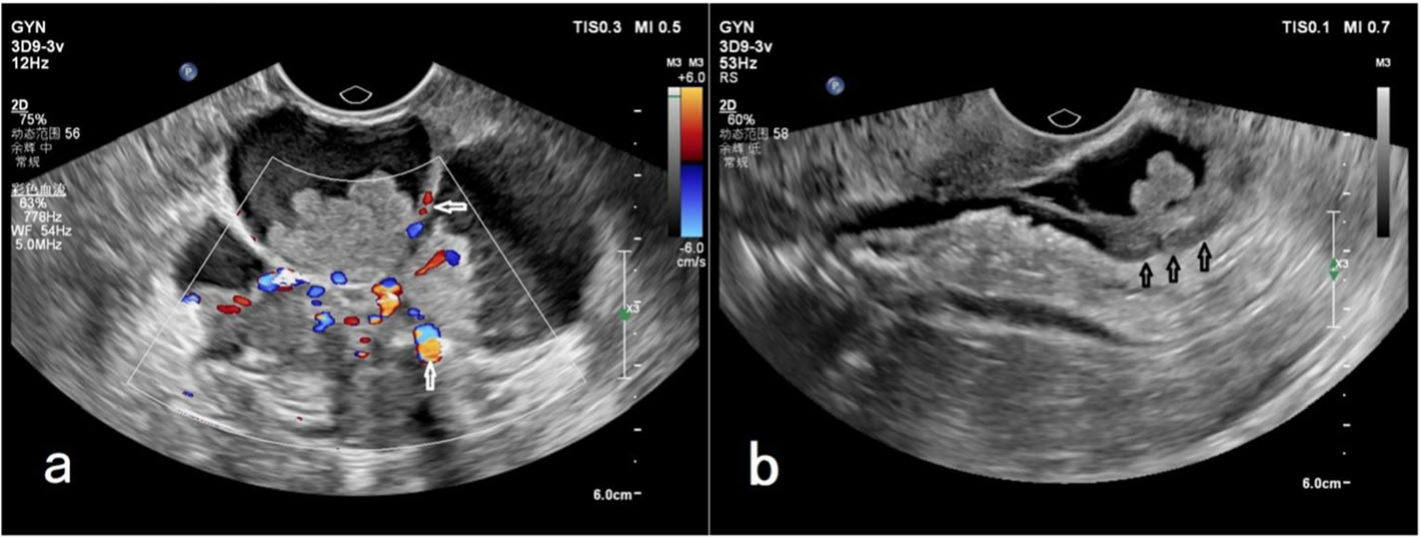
Transvaginal ultrasound (TVA), showing an irregular complex mass in the rectovaginal fossa. a Abundant vascularities on the septa and solid portion of the tumor (arrows). b Invasion of the mass into the anterior wall of the rectum (arrows)
